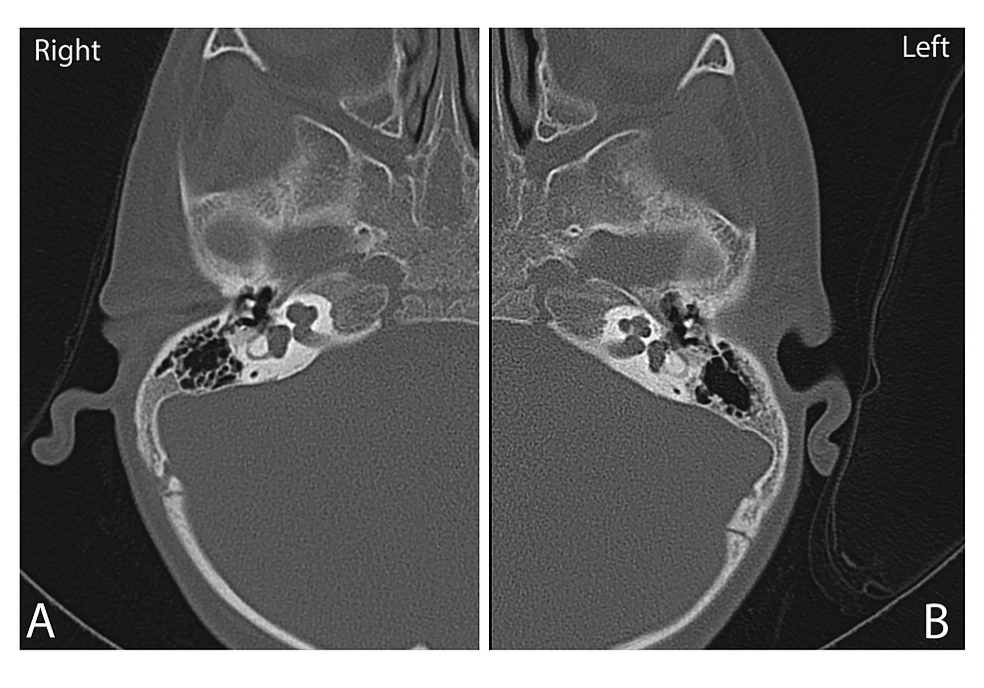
Computed tomography (CT) scan of the inner ears A) CT scan of the right ear shows right cochlear anomaly with incomplete partition type II and enlarged vestibule B) CT scan of left inner ear shows normal appearance of cochlea and modiolus.
